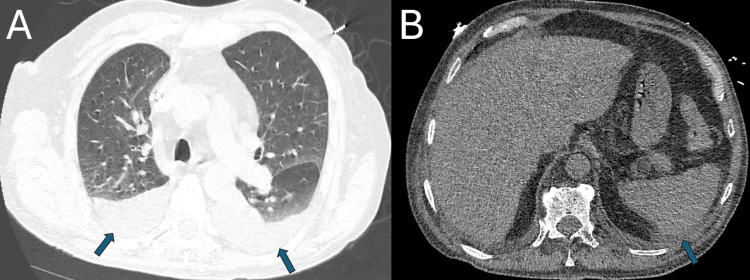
CT imaging without contrast: (A) Moderate bilateral layering pleural effusions (indicated by the blue arrows) and trace interstitial edema. (B) Mild wall thickening and haziness of the peripheral fat, and collection of fluid around the spleen, suggestive of perisplenic ascites (indicated by the blue arrow).
